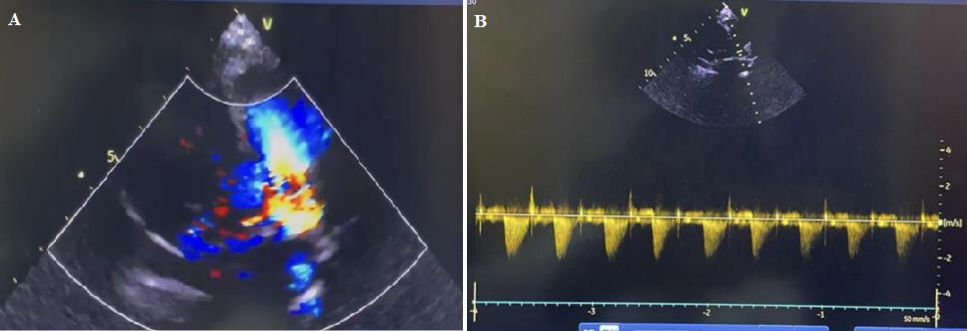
A) color doppler post-PDA ligation showing, no residual PDA flow with non-obstructive supravalvular narrowing; B) post-PDA ligation: good occlusion with no residual flow
